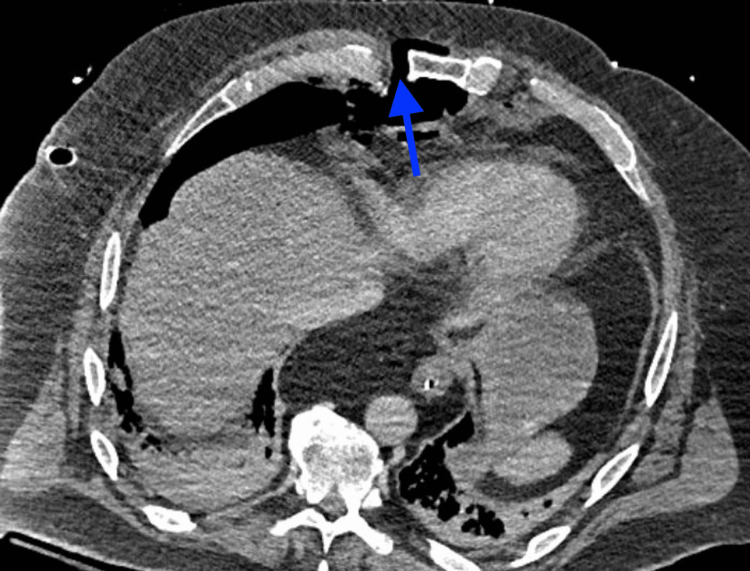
Axial chest CT on day one showing costochondral separation (blue arrow).
